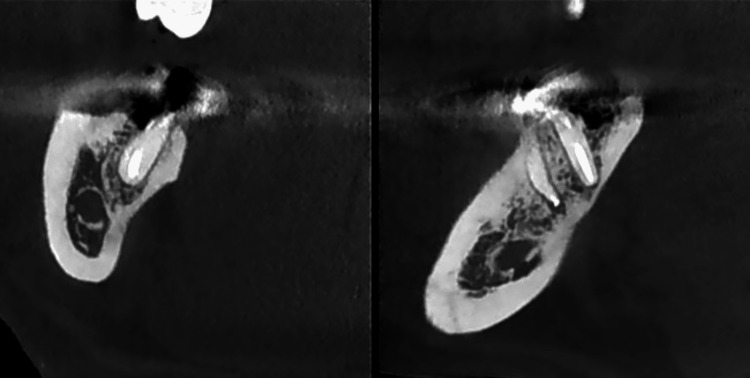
CBCT images of tooth 47, after a three-month period. The left image shows the distal root in the coronal section, while the right image presents the mesial and distal roots in the sagittal section. CBCT, cone beam computed tomography.
